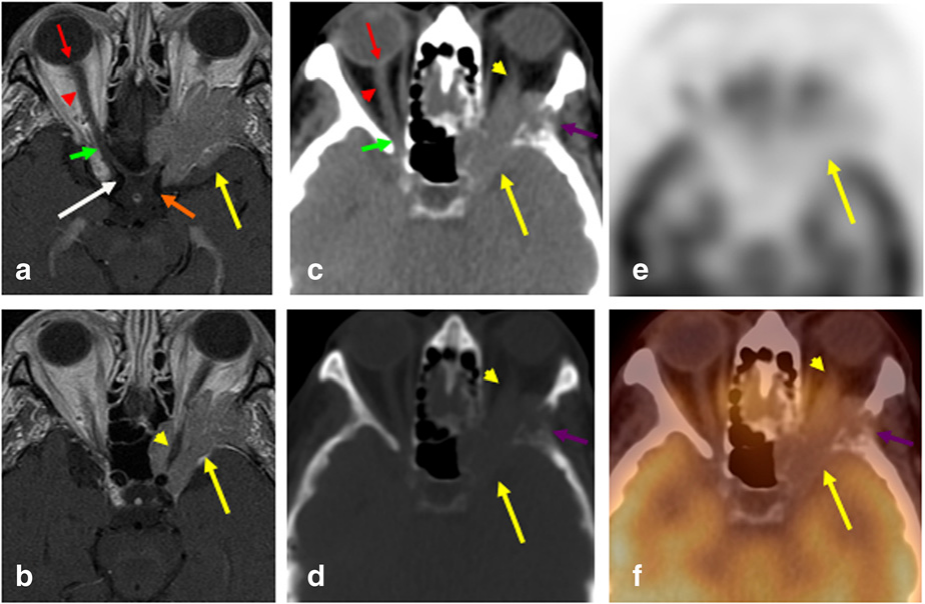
A 74-year-old female complaining of double vision. Axial post contrast T1 WI MRI (a, b), The right optic nerve demonstrates the normal anatomy, showing the four segments of the optic nerve, the retinal (red arrow), orbital (arrow head), canalicular (green arrow) and cisternal parts (white arrow). The optic chiasm is also seen (orange arrow). On the left side, ahomogenously enhancing extra axial mass is seen centered on the eroded left greater wing of thesphenoid bone encasing the left optic nerve. Axial CT soft tissue (c) and bone windows (d), axialPET (e), and fused PET/CT (f) images, showing a hypometabolic soft tissue mass causinghyperostosis and erosion of the left greater wing of the sphenoid and extending into the orbitalcavity along the optic nerve. Diagnosis sphenoid wing meningioma with intra orbital extension,encasing the optic nerve
